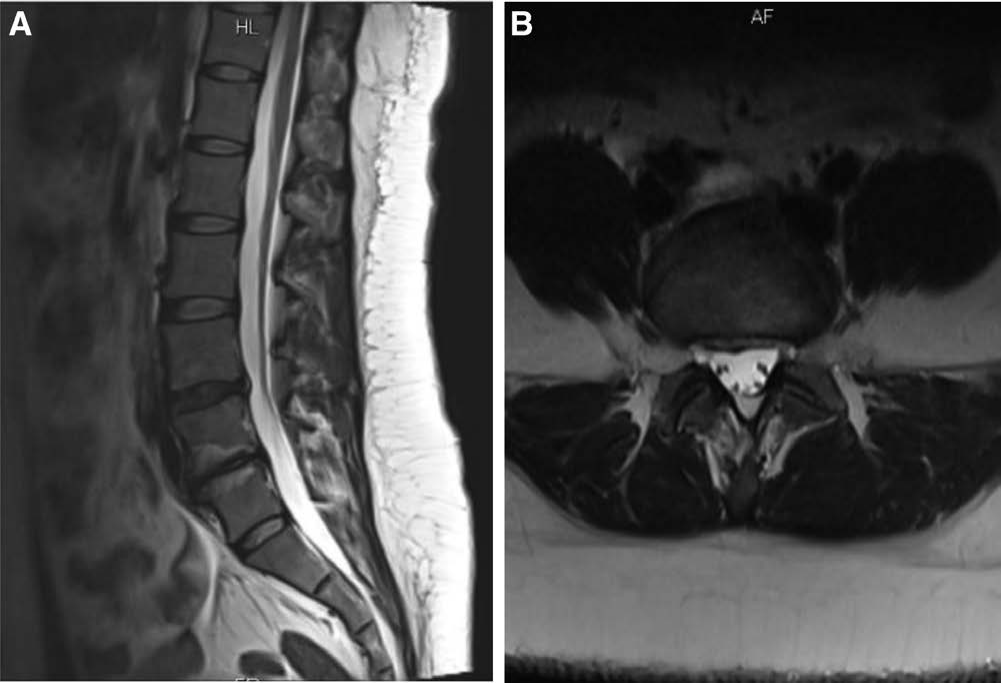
After 12 weeks of treatment, the patient symptoms basically disappeared, and the lumbar MRI scan was reviewed on November 17, 2017: degenerative bulging of the L4/5 disc; degenerative and prolapsed L5/S1 disc; mild lumbar degeneration; sacral lumbarization; L4/5 adjacent vertebral terminal inflammation (Fig. 2A–C).
